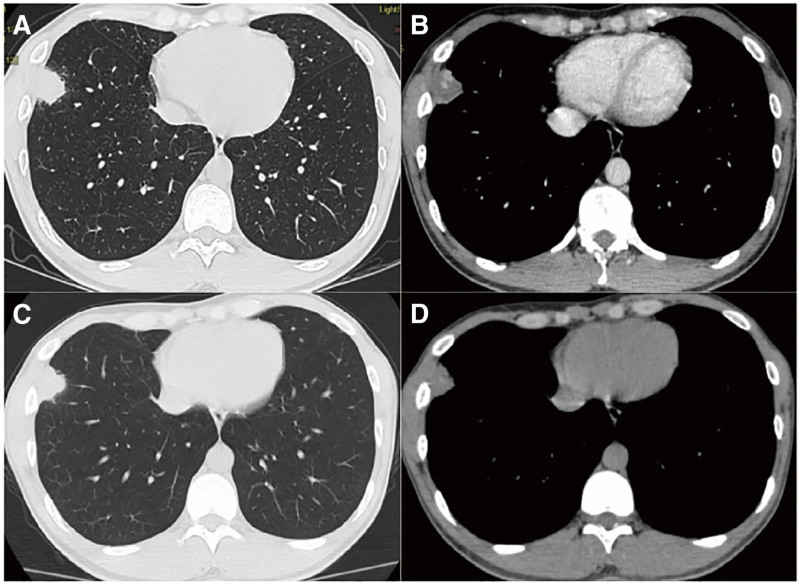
(A, B) Chest enhanced CT of the patient after admission, showing a solid nodule under the pleura of the anterior basal segment of the right lobe. (C, D) CT reexamination of the patient 1 month after antituberculosis treatment, showing that the original lesion has decreased in size.
